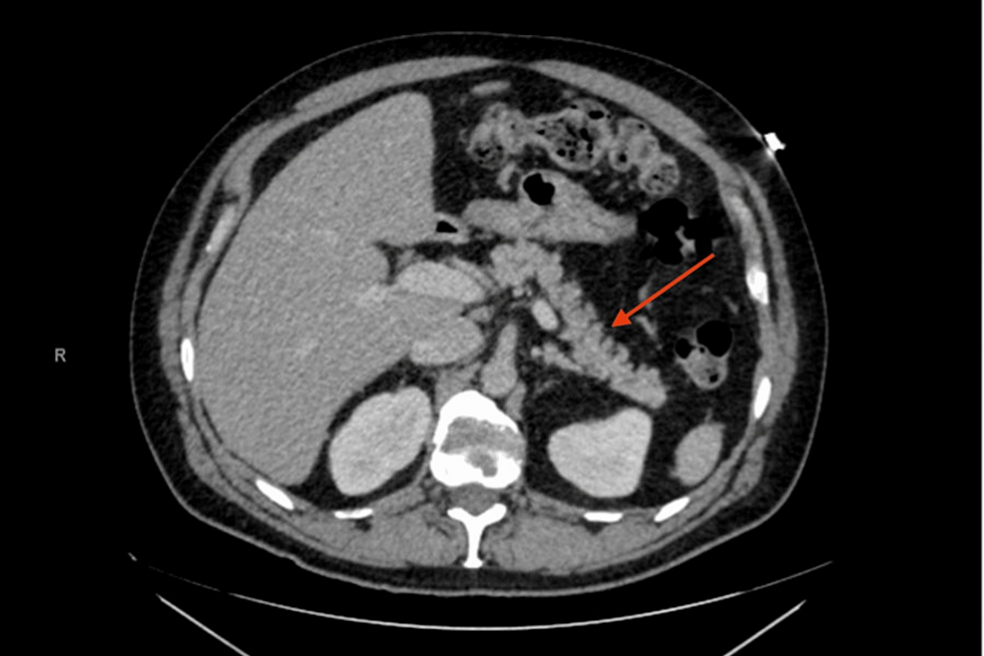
Axial computed tomography scan showing fat stranding along the pancreas head.
